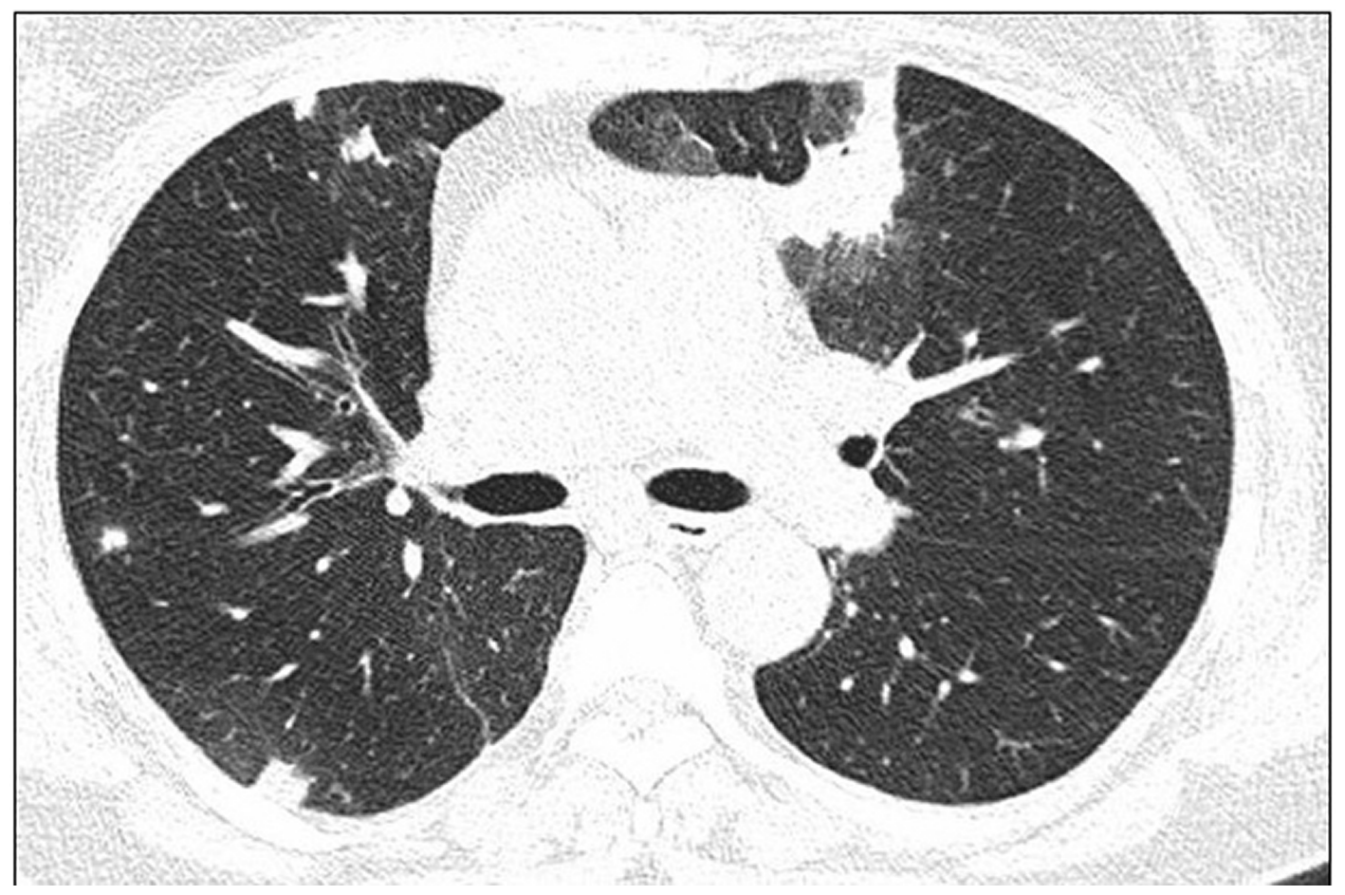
High resolution computed tomography scan of a patient with COP. Bilateral nodular consolidations.
